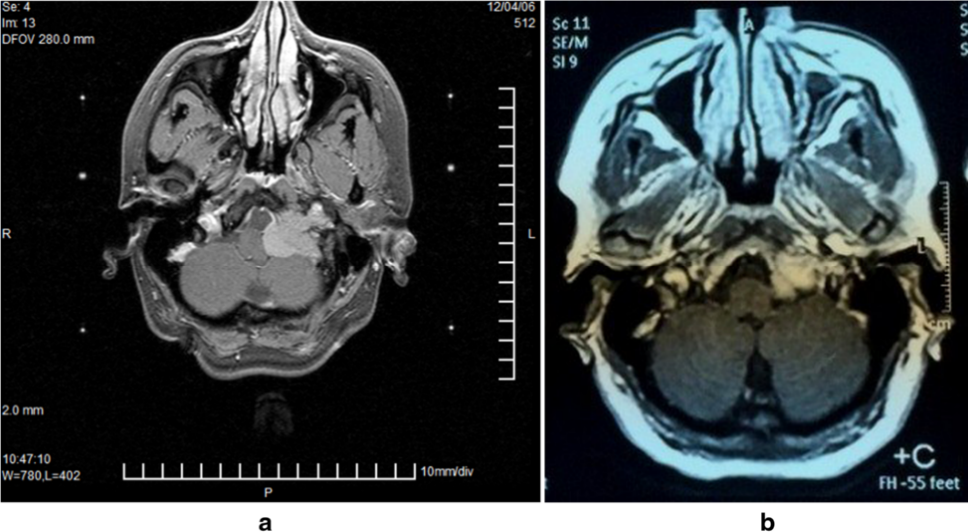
a Pre-gamma knife stereotactic contrast enhanced MR image of a female patient with a large 7.4 cm^3^ left glomus jugulare treated with 15 Gy marginal dose to 35 % isodose line with 98 % coverage with the prescribed dose. b Contrast-enhanced MR image of the same patient 96 months after GKS showing significant tumor regression
